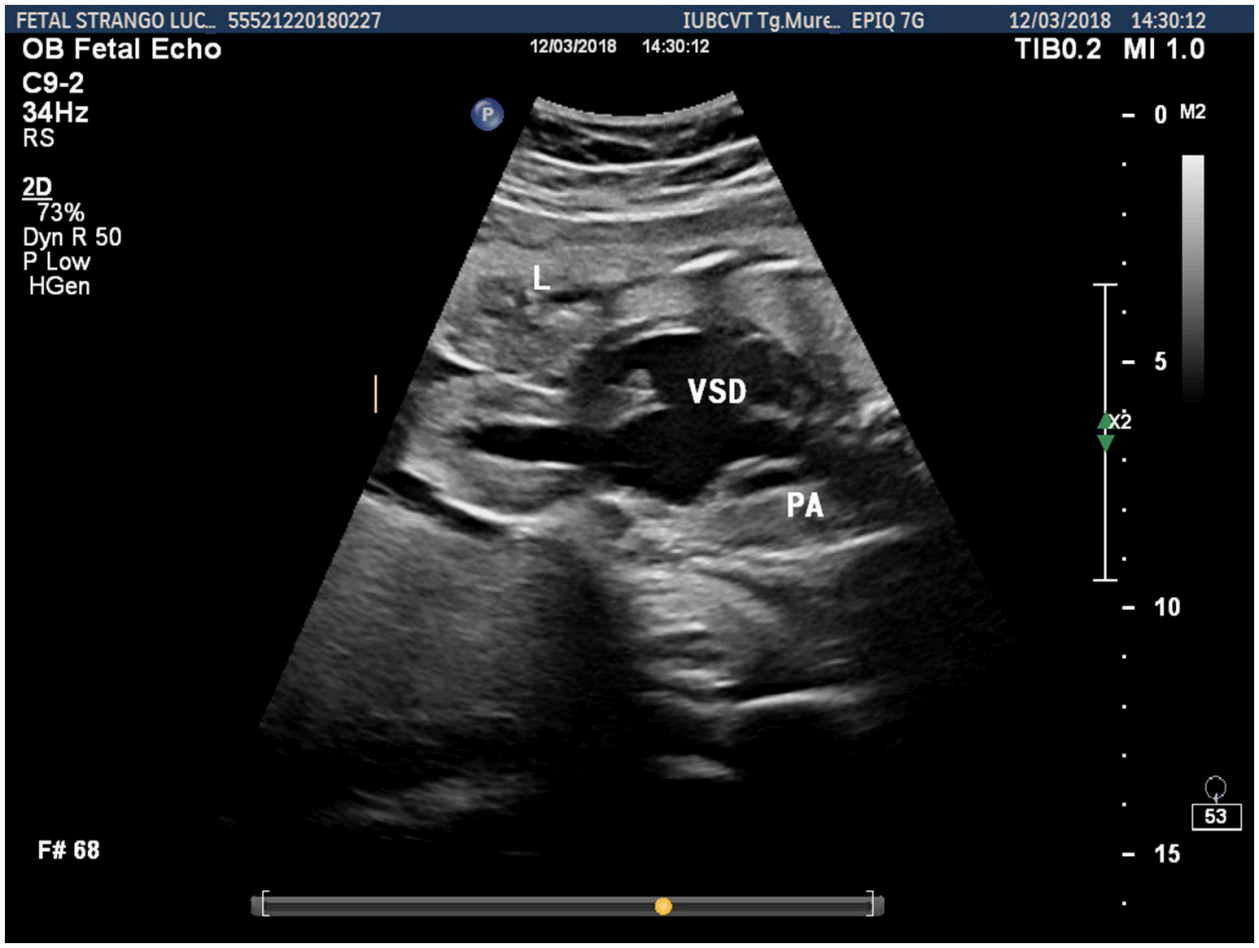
Fetal ultrasonographic image shows an extrathoracic left ventricle, a ventricular septal defect and pulmonary artery hypoplasia (L, liver; VSD, ventricular septal defect; PA, pulmonary artery).
